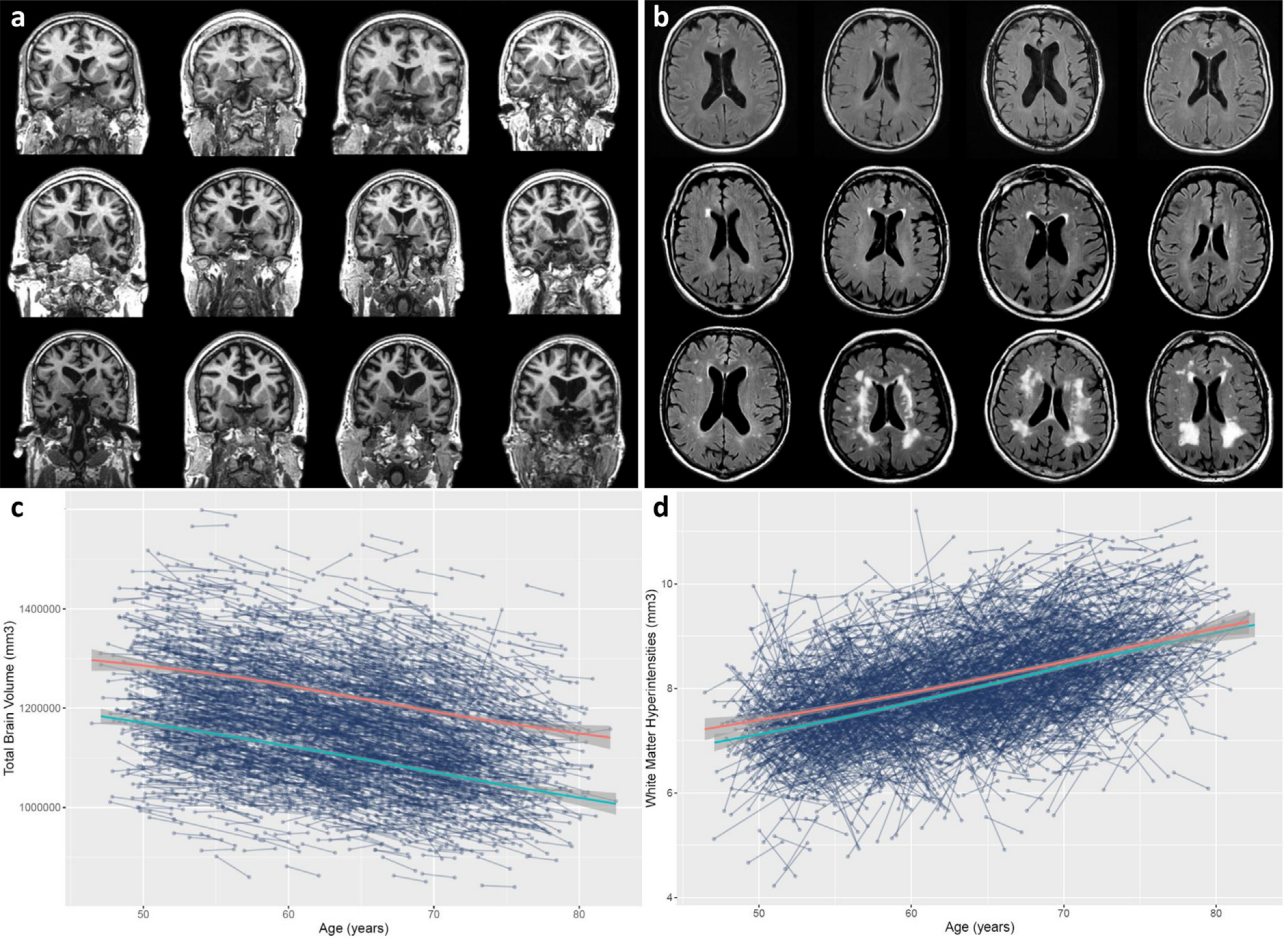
Individual differences in brain structural ageing hallmarks. a) Different participants, ordered from top left to bottom right by severity of global atrophy (parenchymal volume fraction: total brain volume / intracranial volume). MRI-visible markers include global cerebral atrophy, cortical thinning, and ventricular enlargement alongside sulcal widening as CSF (black) replaces brain tissue. b) T2 FLAIR sequence shows increasing severity of white matter hyperinensities (volume increasing from top left to bottom right). Participants from this figure come from the Lothian Birth Cohort 1936 wave 2: community-dwelling adults aged ∼ 73 years at time of scanning. Participants between panels do not correspond. c) Longitudinal total brain volume measurements from 3189 individuals in UK Biobank (Field ID: 20510) with local polynomial regression lines (loess) for females (red) and males (blue). d) Longitudinal white matter hyperintensity volume measurements from 3152 individuals in UKB Biobank (Field ID: 25781) with local polynomial regression lines (loess) for females (red) and males (blue). (For interpretation of the references to colour in this figure legend, the reader is referred to the web version of this article.)
